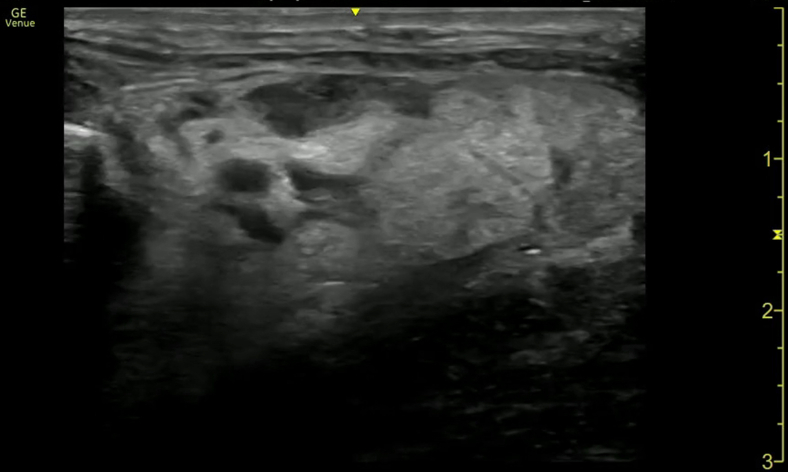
Point-of-care ultrasound with a linear probe showing the transverse view of the submandibular neck. There is cobblestoning of the subcutaneous tissue with subcutaneous fluid collections, an enlarged lymph node, and sonographic evidence of abscess.
